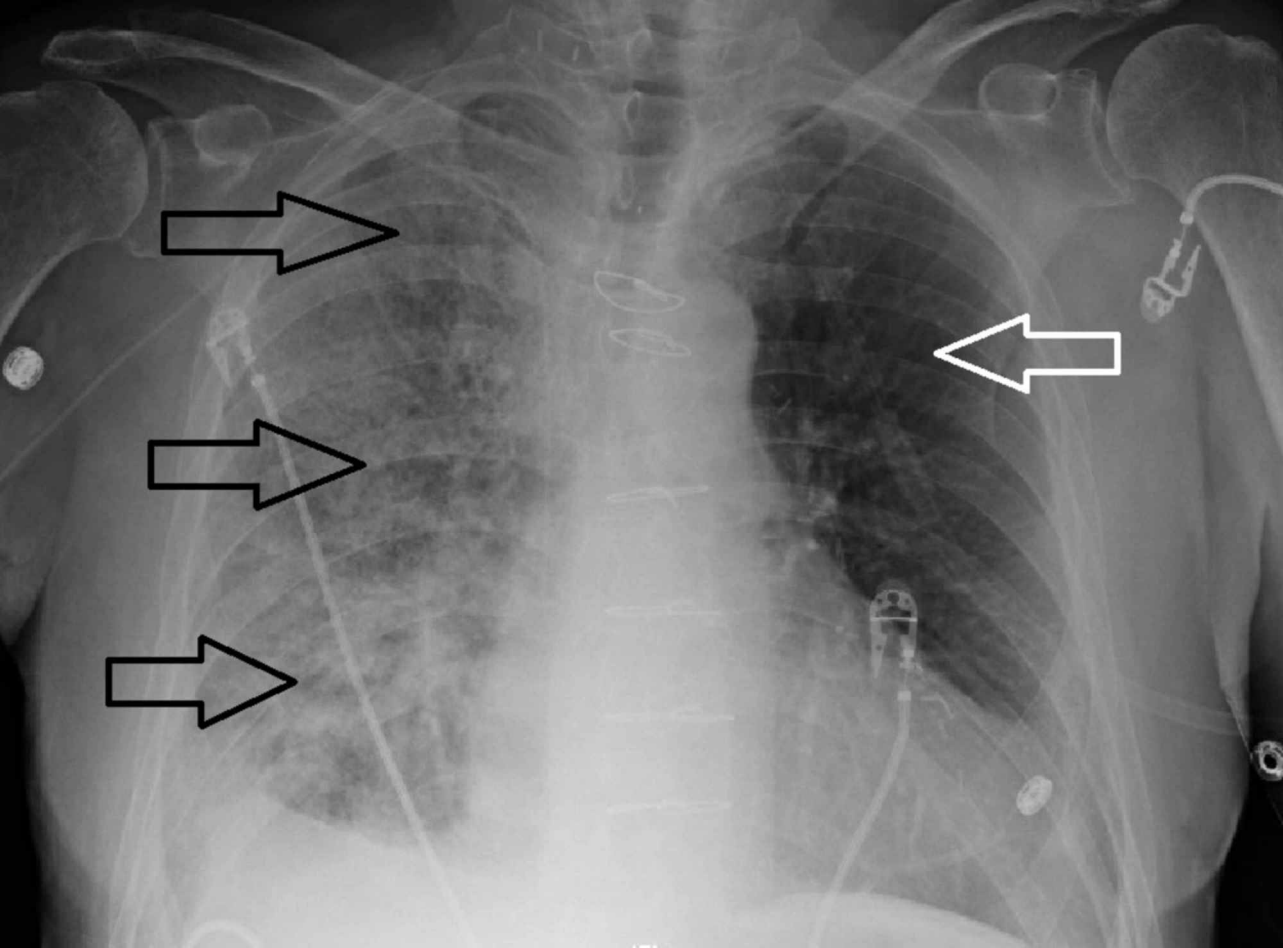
Portable chest X-ray demonstrating multifocal opacities involving all lobes of right lung (black arrows). Left lung parenchyma is relatively preserved (white arrow).
